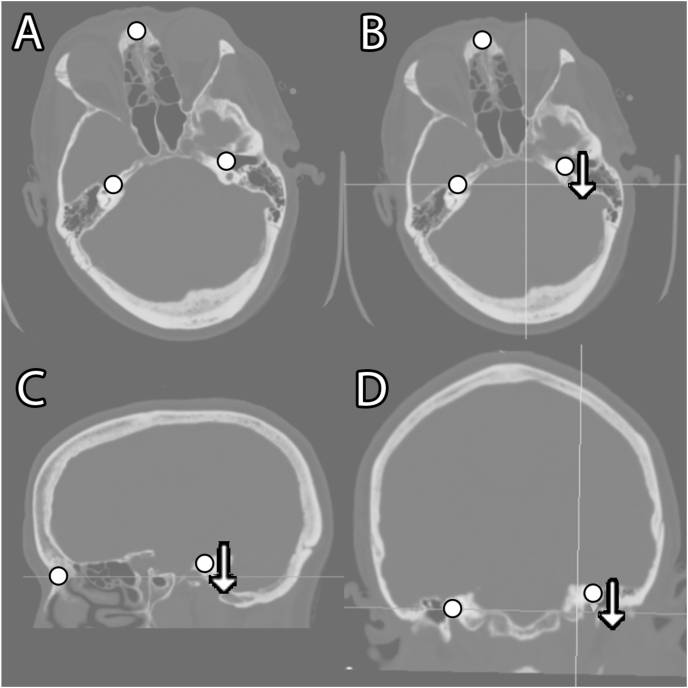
Landmark alignment technique. CT images are shown in a bone window setting to better demonstrate skull base structures. Initial CT (A) is shown in a plane including all three landmarks (black circles on nasal bridge, right cochlea, left cochlea). First, axial rotation to align the cochleas on the y axis (B), then sagittal rotation to align the nasal bridge and right cochlea on the z axis (C), then coronal rotation to align the cochleas on the z axis (D).
